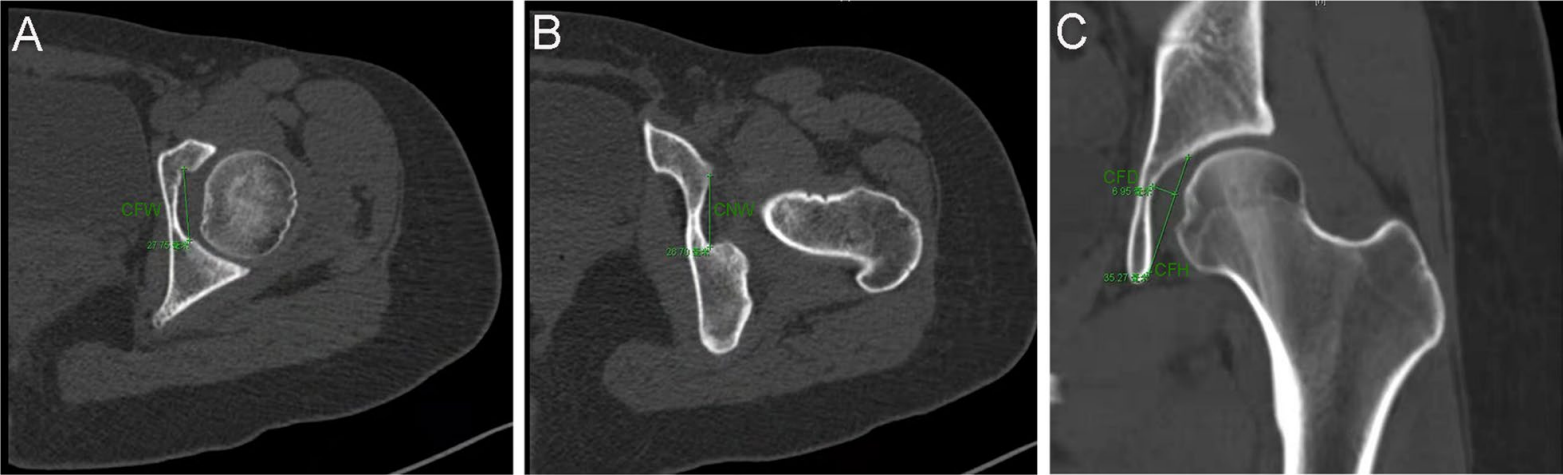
The measurement of the linear length of cotyloid fossa on CT images of the left hip. a and b CFW and CNW were measured on axial images; c CFH and CFD were measured on coronal images. CFW = Cotyloid Fossa Width; CFH = Cotyloid Fossa Height; CFD = Cotyloid Fossa Depth; CNW = cotyloid notch Width
